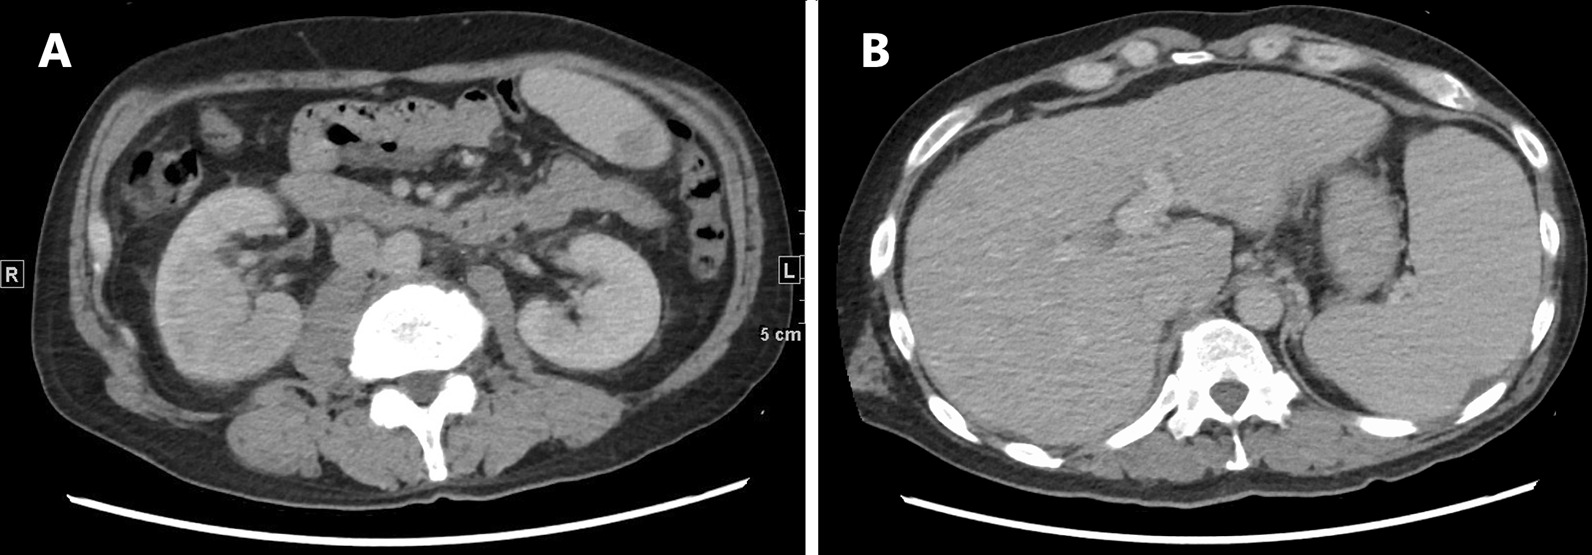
Contrast-enhanced computed tomographic images revealing heterogeneous enhancement of both kidneys in A, and a liver with a blunt edge and irregular surface in B
